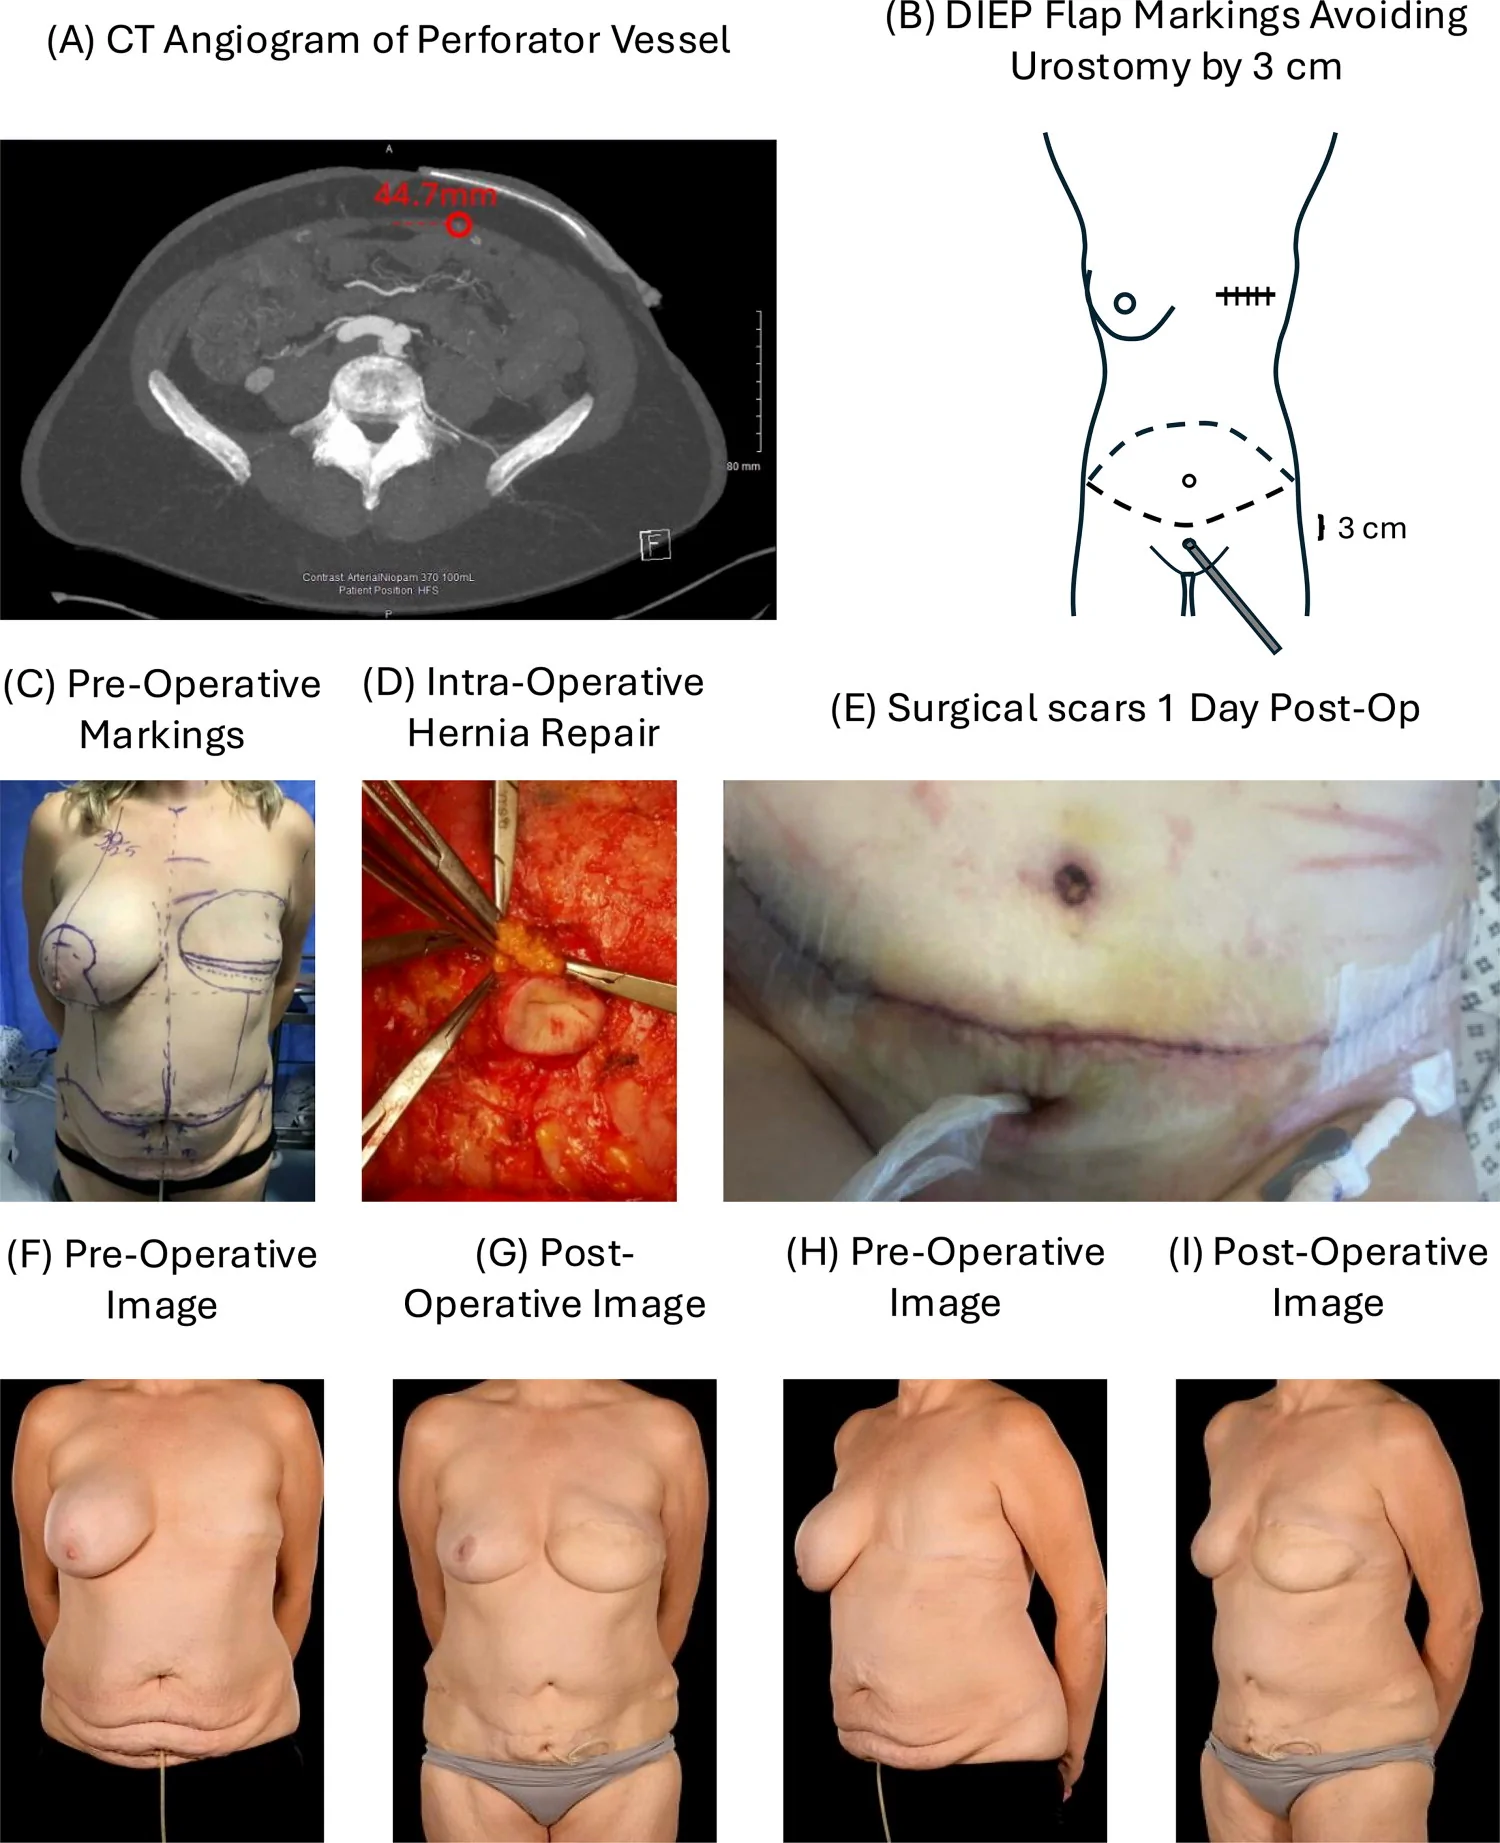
(A) Pre-operative CT Angiogram identified three viable perforators, one of which is shown. (B) DIEP flap markings indicating position of urostomy. Markings avoided urostomy by about three centimetres. (C) Pre-operative appearance of mastectomy deformity and significant size and shape asymmetry of the contralateral breast. Markings indicative DIEP flap harvest location, transfer location and mastopexy markings. (D) Intra-operative incidental hernia repair. (E) Donor site scar, one day post-op with a suprapubic catheter *in situ*. (F, H) Pre-operative images showing a previous left mastectomy and implant-augmented right breast complicated by capsular contracture. There is an adequate abdominal pannus despite the pre-existing scarring. (G, I) Post-operative appearances 16 months later demonstrating the soft supple reconstructed left breast with a recreated inframammary fold. The uplifted and explanted right breast facilitated implant achievement of volume and shape symmetry.
